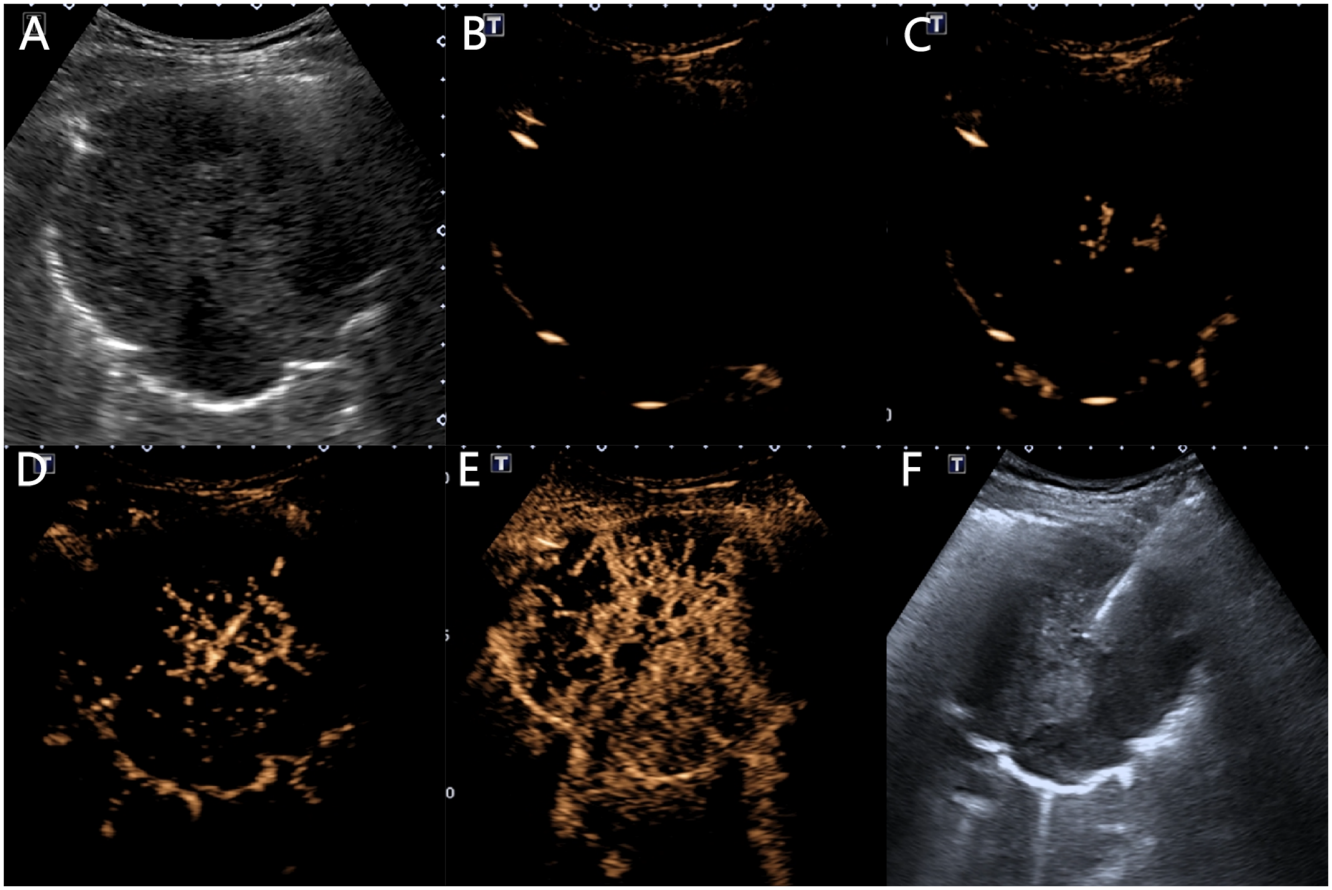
Ultrasound manifestations of a 64-year-old male patient with squamous cell carcinoma. (A) A low-echo solid mass of 10.1 × 8.3 cm can be seen in the right lung, with a circular shape and clear boundaries. (B) At 2.8 s, the lung tissue begins to enhance with inflation. (C) At 7.6 s, the lesion begins to centrifugally enhance in a patchy manner. (D) At 8.8 s, the chest wall begins to enhance. (E) The lesion shows heterogeneous low enhancement, with multiple necrotic areas visible inside, and the enhanced morphology is sieve-like. (F) A 16G puncture needle is used to puncture and sample the central non-necrotic area of the lesion.
